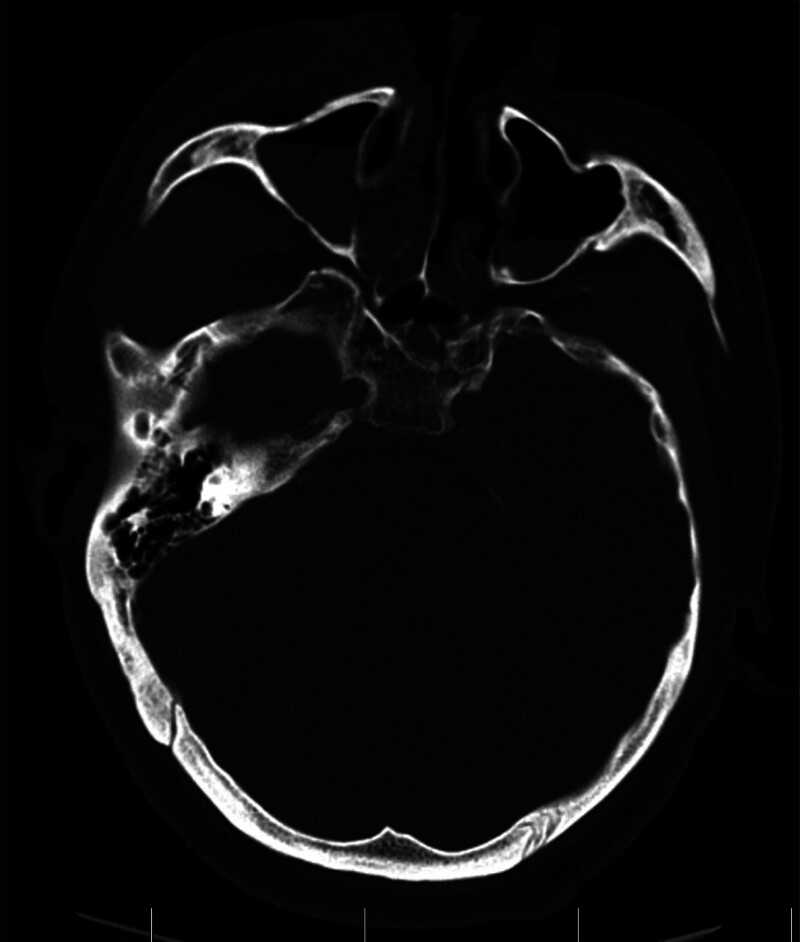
On August 2nd, 1 year ago, CT images of the patient showed fracture lines in the frontal bone, maxilla and sphenoid bone, which involved the inner wall of the right orbit and showed high-density shadows in the sinus cavity. CT = computed tomography.
